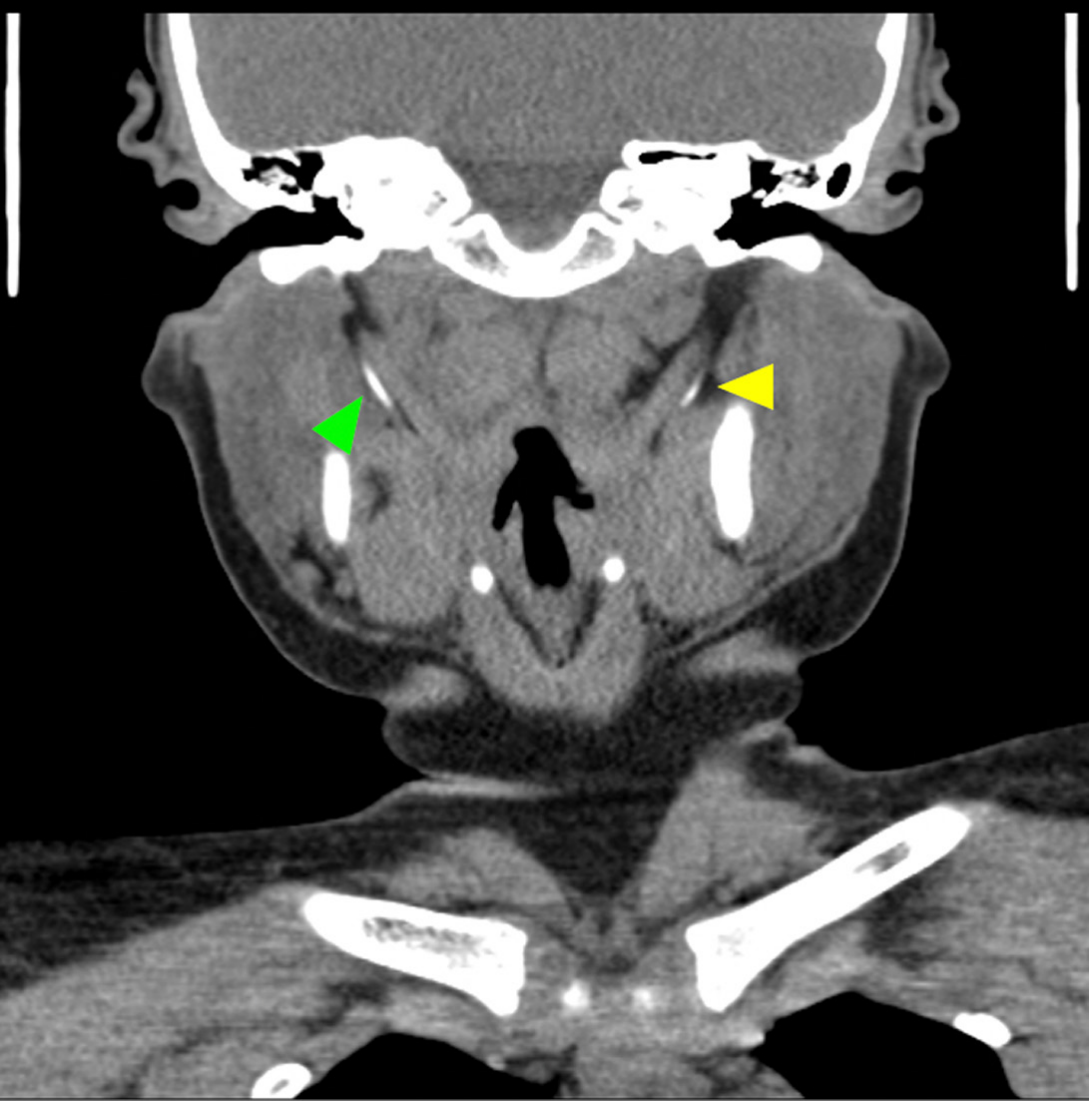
Coronal computed tomography (CT) without contrast revealing bilateral elongated styloid processes
